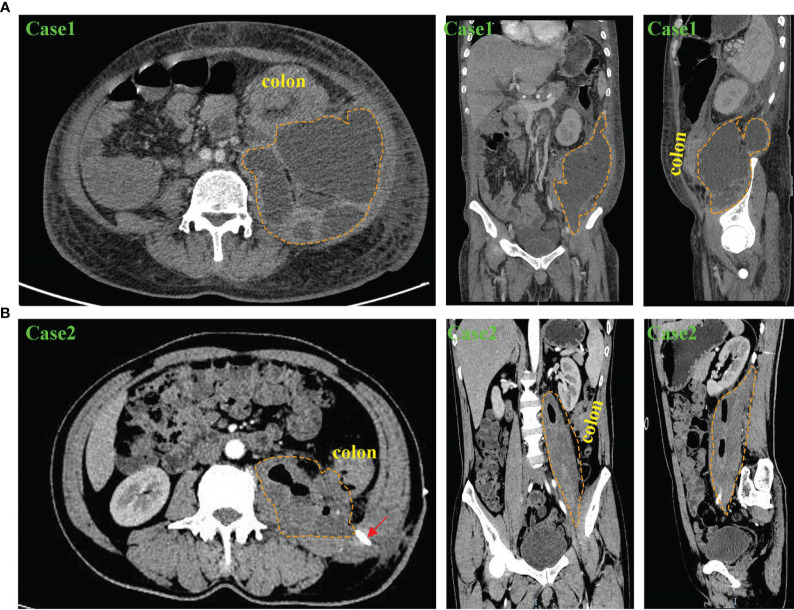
Abdominal and pelvic CT scan results of patients with retroperitoneal abscess in our department. (A) CT findings of Case 1 in transverse, coronal and sagittal planes. The results showed a huge right retroperitoneal and iliopsoas abscess with thickening of the descending colon wall. The yellow dashed circle shows the extent of the abscess. (B) CT findings of Case 2 in transverse, coronal and sagittal planes. The results showed a right retroperitoneal iliopsoas abscess and thickening of the descending colon wall. The yellow dashed circle shows the extent of the abscess, and the red arrow shows the drainage tube for percutaneous drainage.
